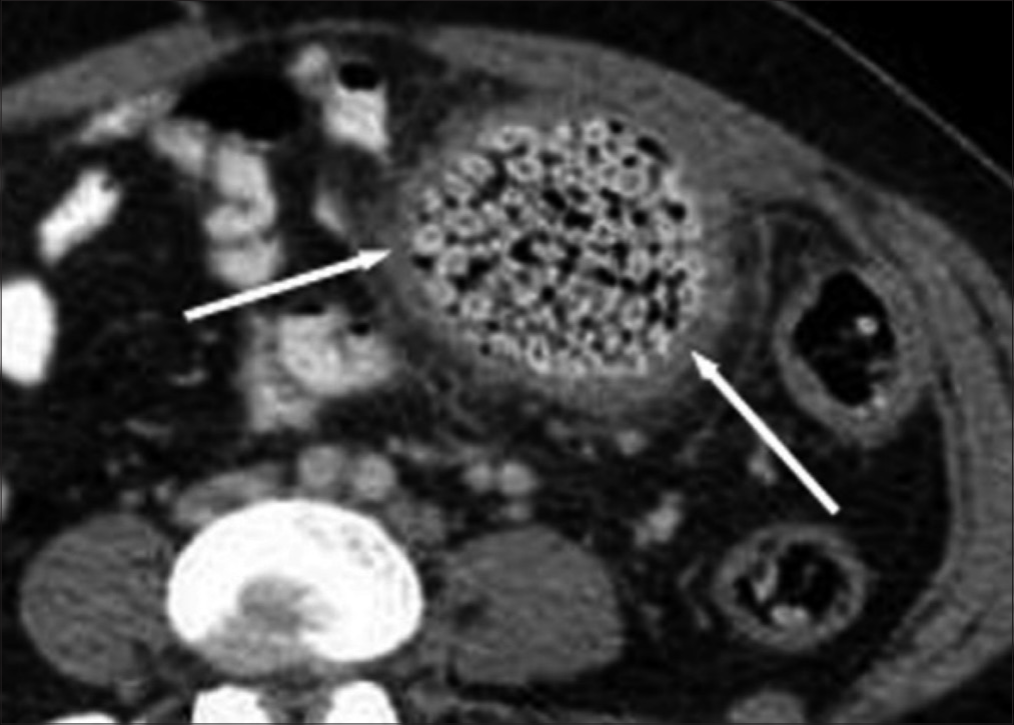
Axial contrast-enhanced CT scan showing the sigmoid colon seed bezoar along with the inflammatory wall thickening (arrows)
